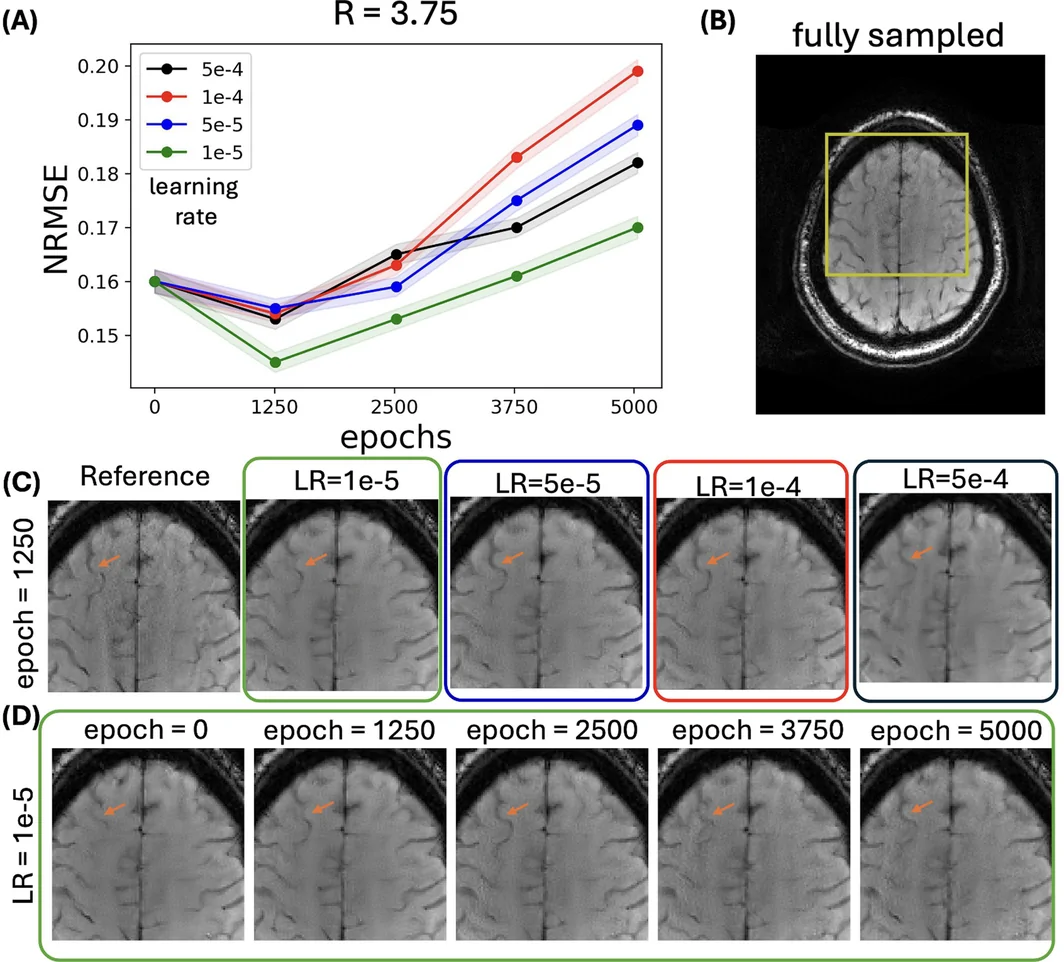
(A) NRMSE of SWI reconstructions averaged over five validation subjects from data retrospectively accelerated by R≈3.75. (B) Fully‐sampled reference. (C) Zoomed‐in reconstructions obtained with fixed epochs and varying learning rate and (D) fixed learning rate and varying epochs. Similar to the fastMRI FLAIR experiments, insufficient or excessive fine‐tuning duration and higher learning rates consistently result in worse reconstructions, demonstrating that the proposed training strategy is effective for clinical stroke SWI data.
